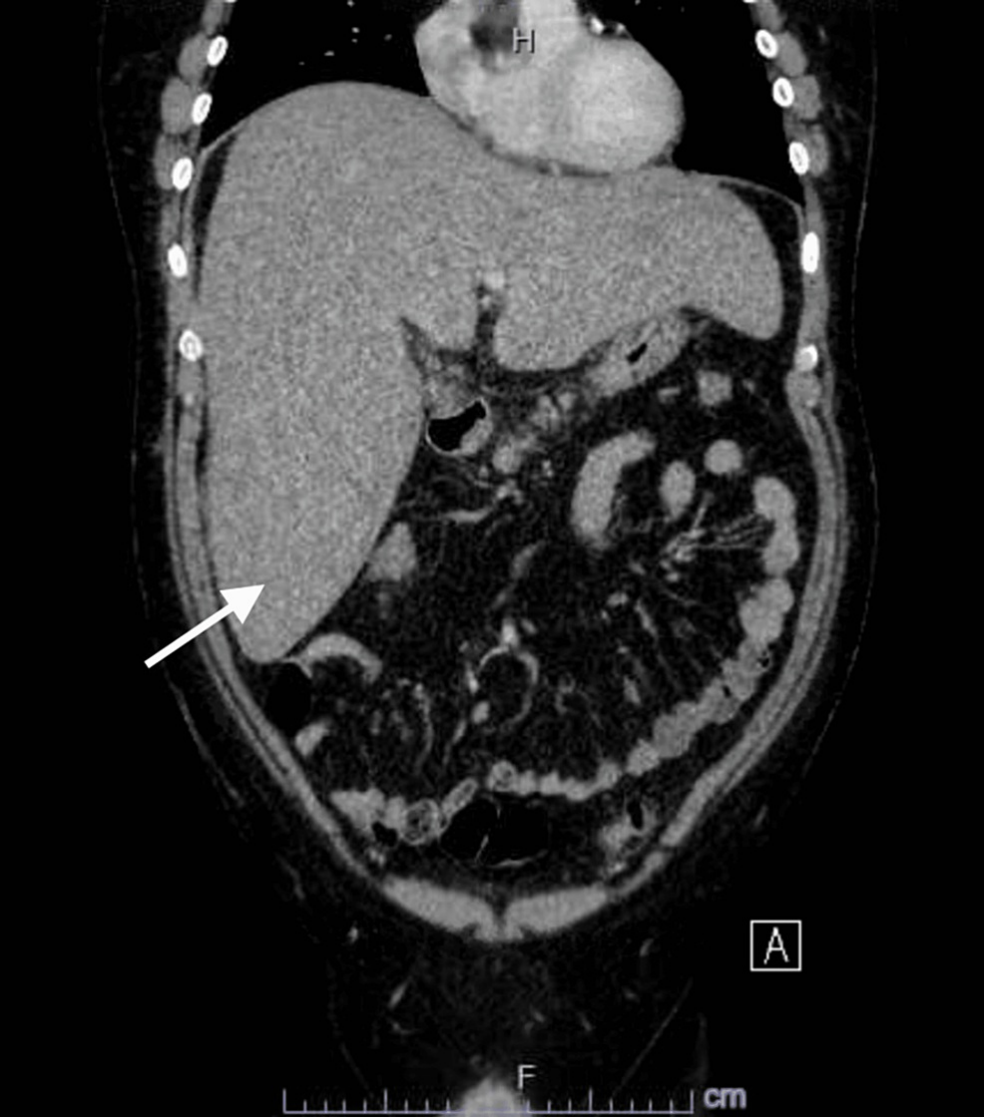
Coronal CT scan of the abdomen Coronal computed tomography (CT) scan of the abdomen showing evidence of hepatomegaly (arrow).
